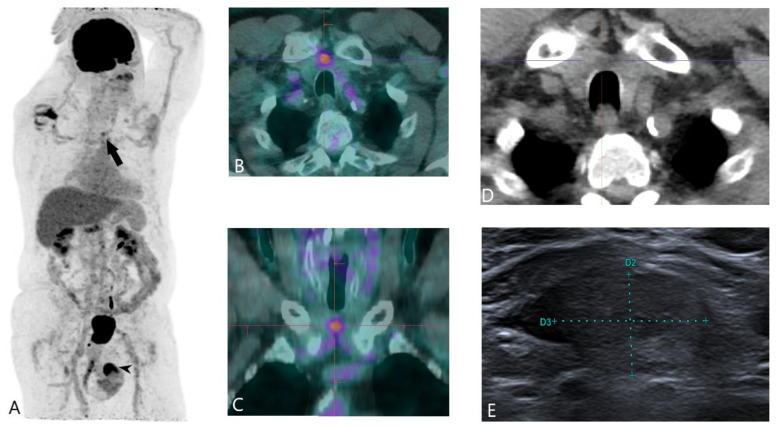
Incidental FDG-avid nodule in the thyroid isthmus discovered during a staging PET/CT study for penile cancer. Fine-needle aspiration confirmed papillary thyroid carcinoma. (A) Maximum intensity projection (MIP) of the FDG PET/CT. The black arrow shows a moderate hypermetabolic focus in the thyroid isthmus, and the black arrowhead denotes an intense hypermetabolic focus associated with penile cancer. (B) Axial fused and (C) coronal fused FDG PET/CT images showing the hypermetabolic nodule in the thyroid isthmus. (D) Axial CT image displaying a supra-centimetric, hypodense nodule. (E) Ultrasound image showing the nodule measuring 17 × 12 mm.
